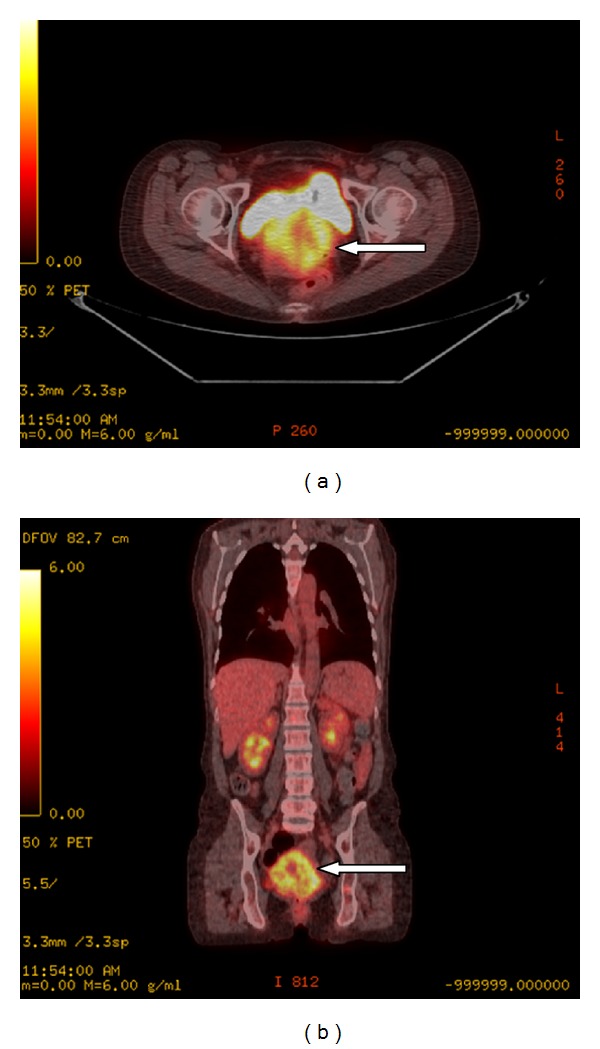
Axial (a) and coronal (b) PET-CT fusion images of the pelvis demonstrate a biopsy-proven lymphomatous 8.4 cm × 5.3 cm mass in the cervix (arrow) with a maximum SUV of 20.7.
